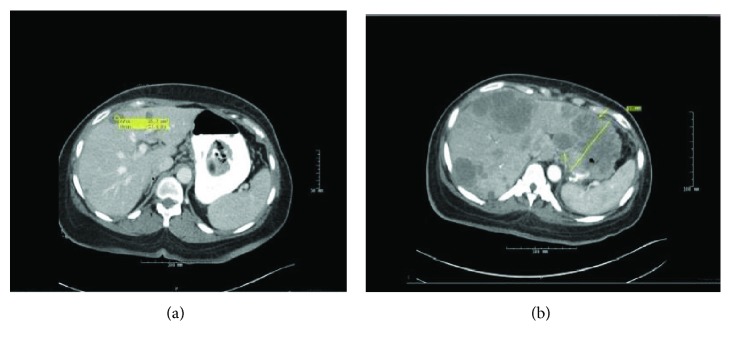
(a) CT imaging of the abdomen and pelvis with IV and oral contrast. Initial presentation with gastric mass and liver metastasis. (b) Repeated imaging on subsequent admission 6 weeks later with an increase in the size of gastric mass and liver invasion and worsening metastasis.
